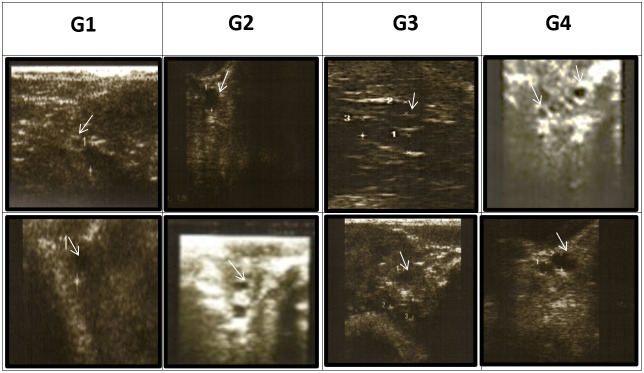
Effect of treatment of CSFA supplementation and/or superovulation with hormonal treatment on the activity of the ovaries of Barki ewes by using ultrasonography. Numerous follicles are between 3 and 5 mm in diameter. (A) First-type diameter of small follicles (
